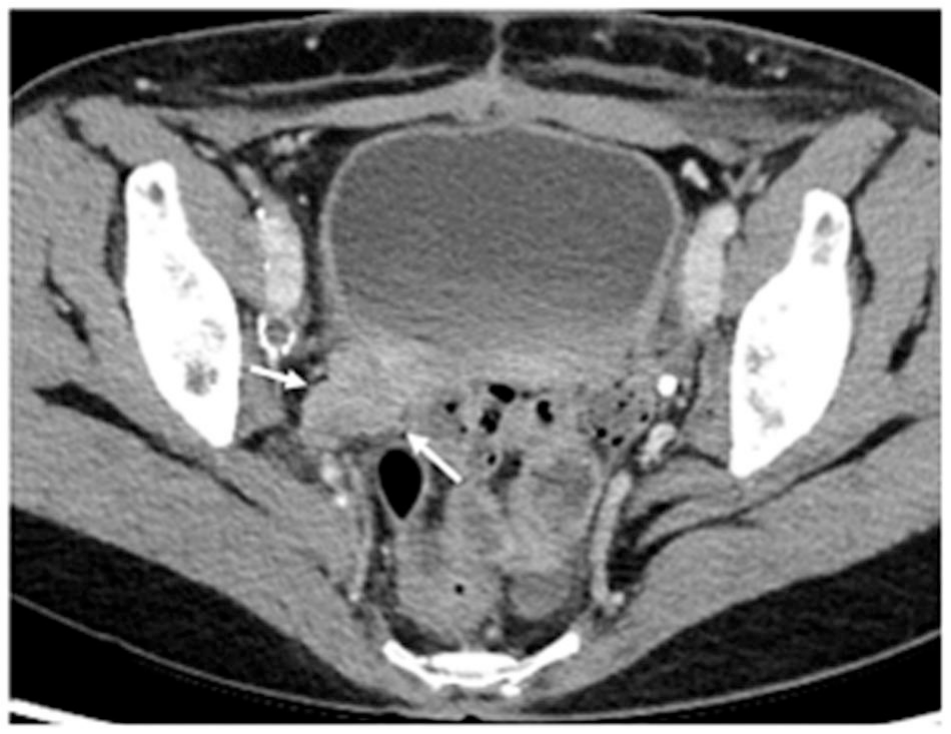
Contrast-enhanced CT at 10 months postoperatively. A 1.5 cm mass is observed on the right side of the pelvic cavity, with suspicion of disseminated recurrence (arrow).
